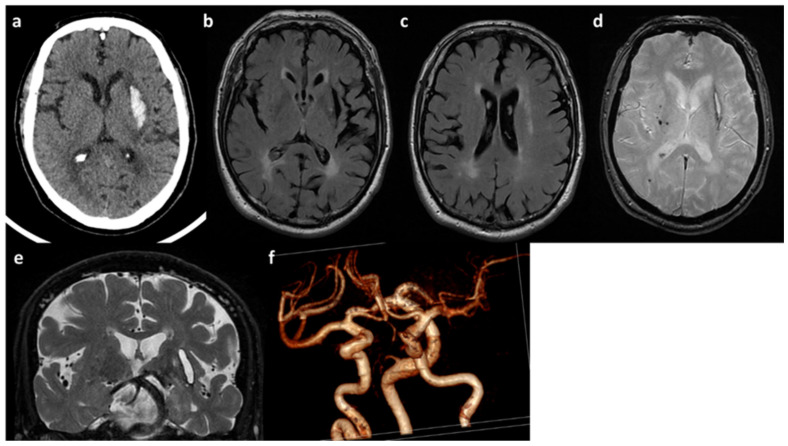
Neuroimaging features of patient B. (a) Brain CT with acute left ICH in the external capsule. (b,c) Axial FLAIR sequences of brain MRI with leukoaraiosis and cortical atrophy. (d) T2* sequence of brain MRI with lobar microbleeds. (e) Coronal T2W sequence of brain MRI with enlarged perivascular spaces and dolichoectatic basilar artery. (f) MRA (3D reconstruction) showing the main intracranial arteries with dilatative arteriopathy.
